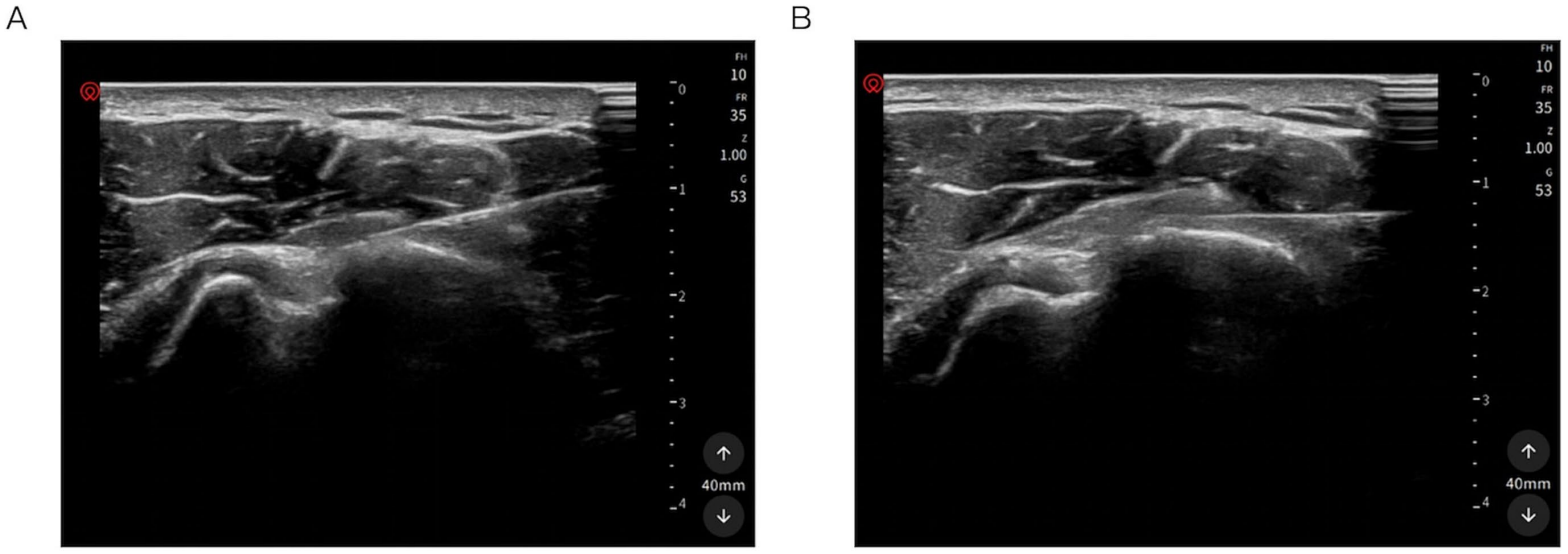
Ultrasound-guided PRP injection. (A) Injection with the shoulder in the neutral position. (B) External rotation of the shoulder to optimize the spread of the injectate within the injured tendon.
